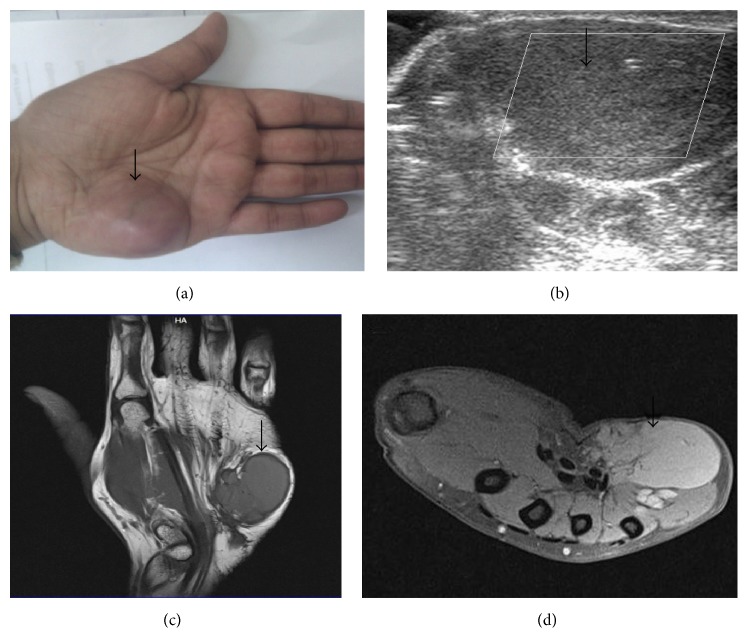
Synovial sarcoma. A 34-year-old female patient presented with noncompressible swelling involving hypothenar eminence of left hand ((a), arrow). Ultrasonography shows a hypoechoic lesion with absent color flow (b). MRI shows a large, lobulated T1W hypointense ((c), arrow) and T1WFS hyperintense ((d), arrow) lesion.
